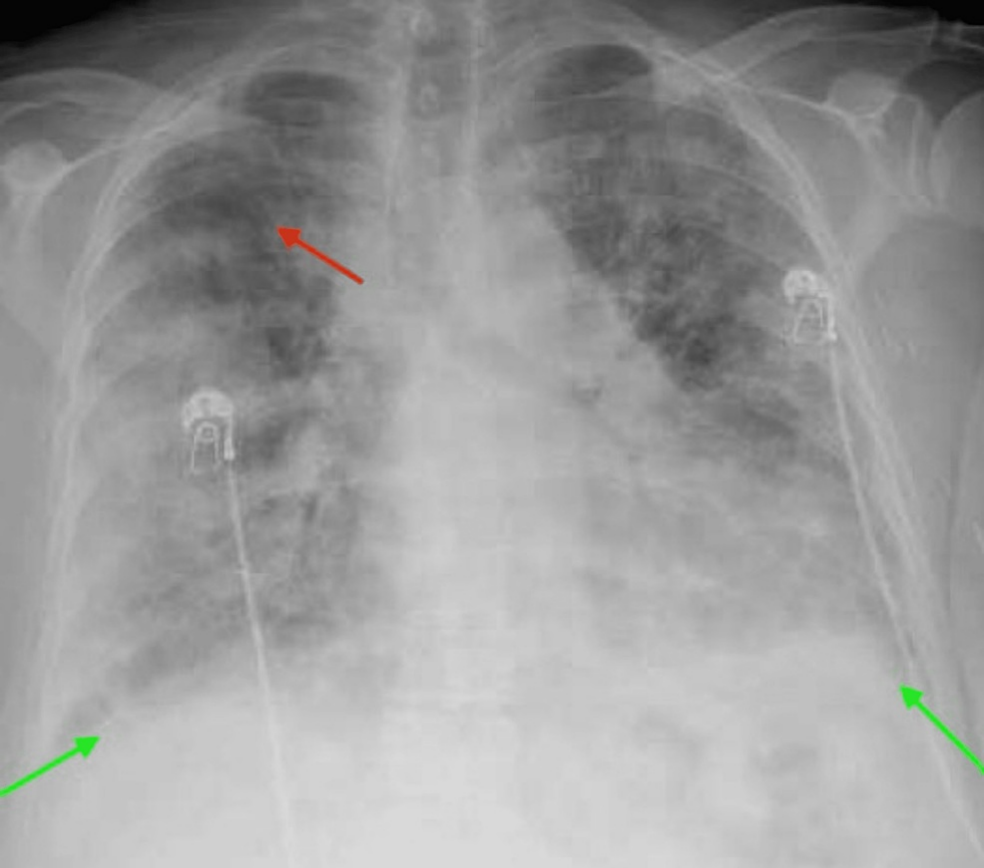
Chest X-ray showing ground-glass opacity diffuse patchy airspace disease compatible with congestion, infection, or ARDS. Red arrow shows cephalization of pulmonary vasculature, green arrow shows blunder costophrenic angles bilaterally. ARDS: acute respiratory distress syndrome
